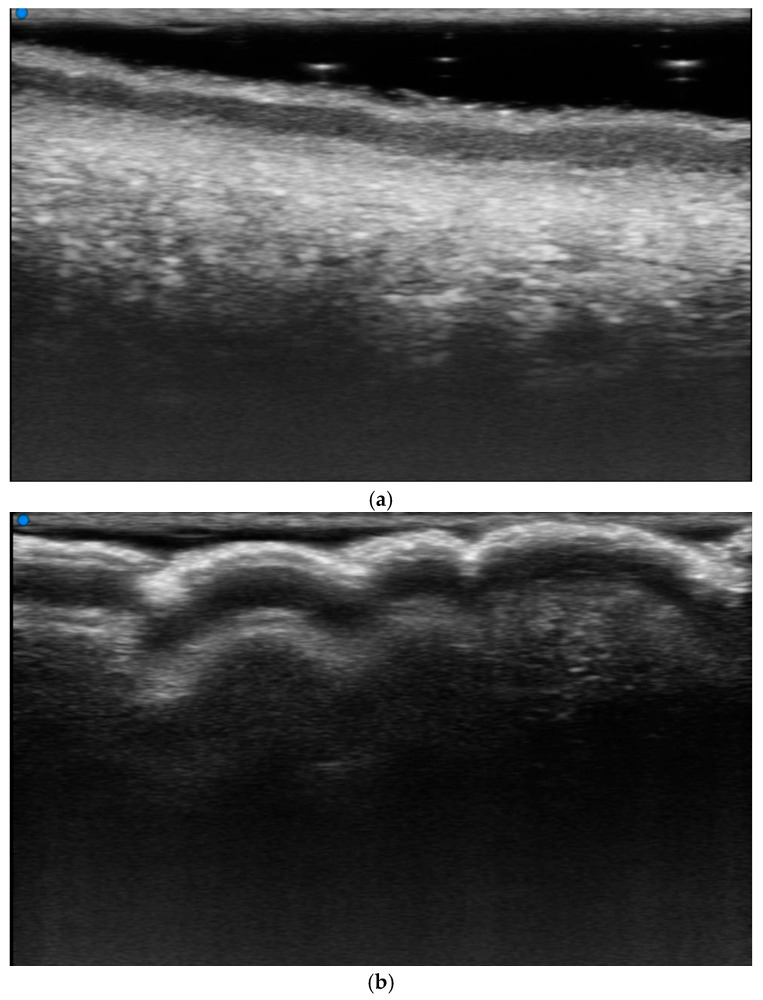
High-frequency ultrasound features of psoriatic plaques: (a) subepidermal low-echogenic band (SLEB) associated with dermal thickening, suggestive of active inflammation; (b) irregular, undulated epidermal surface corresponding to hyperkeratosis and acanthosis, with underlying dermal changes (UHF 70 MHz).
